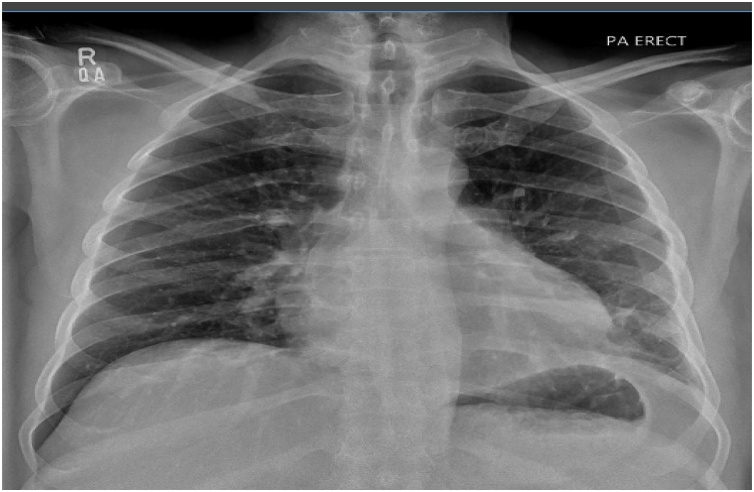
Chest X-ray six month Postoperative.
